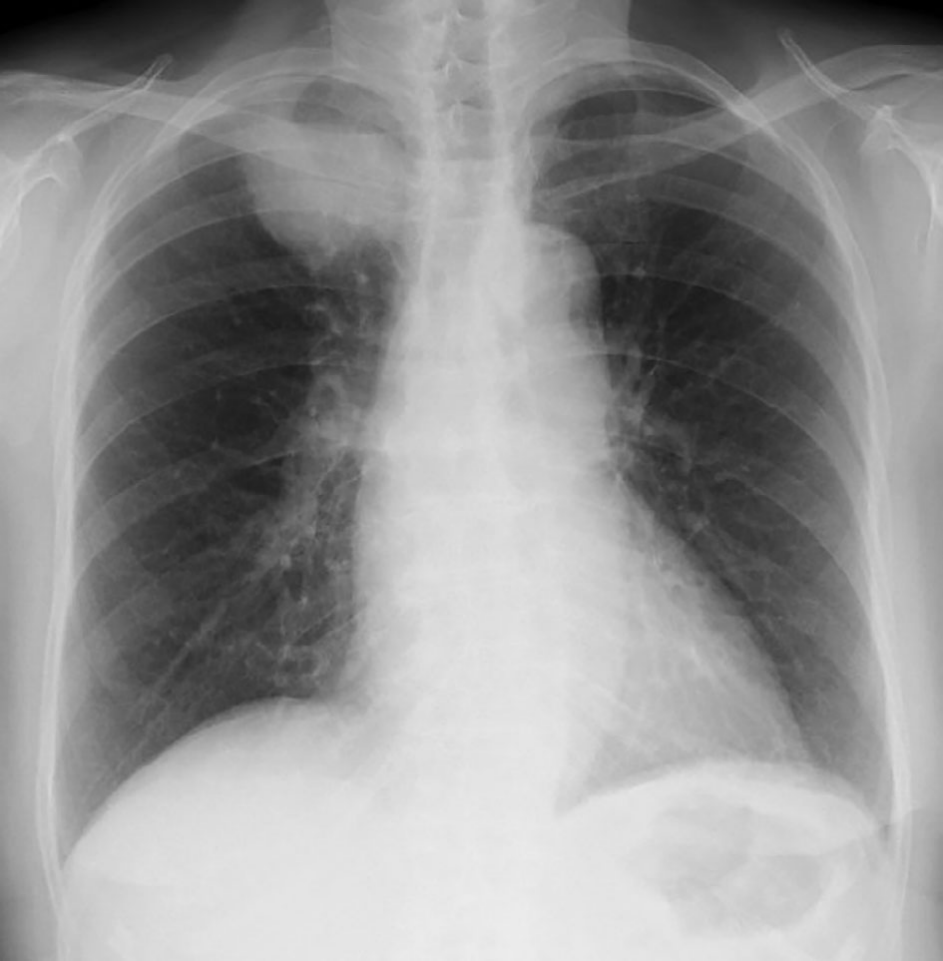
Chest radiograph at hospitalization showed a lung mass in the right upper field.
